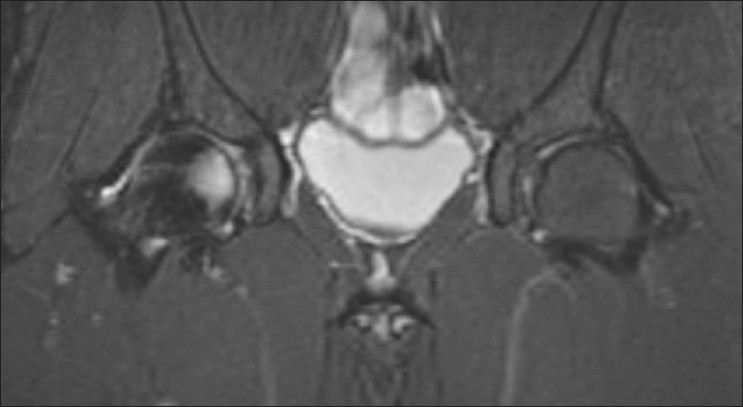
MRI three months after injury. The coronal fast spin echo T2-weighted image with a fat saturation image shows slight increase in size and signal intensity of the right femoral head abnormality. (Images obtained on a 1.5T General Electric MR scanner)
